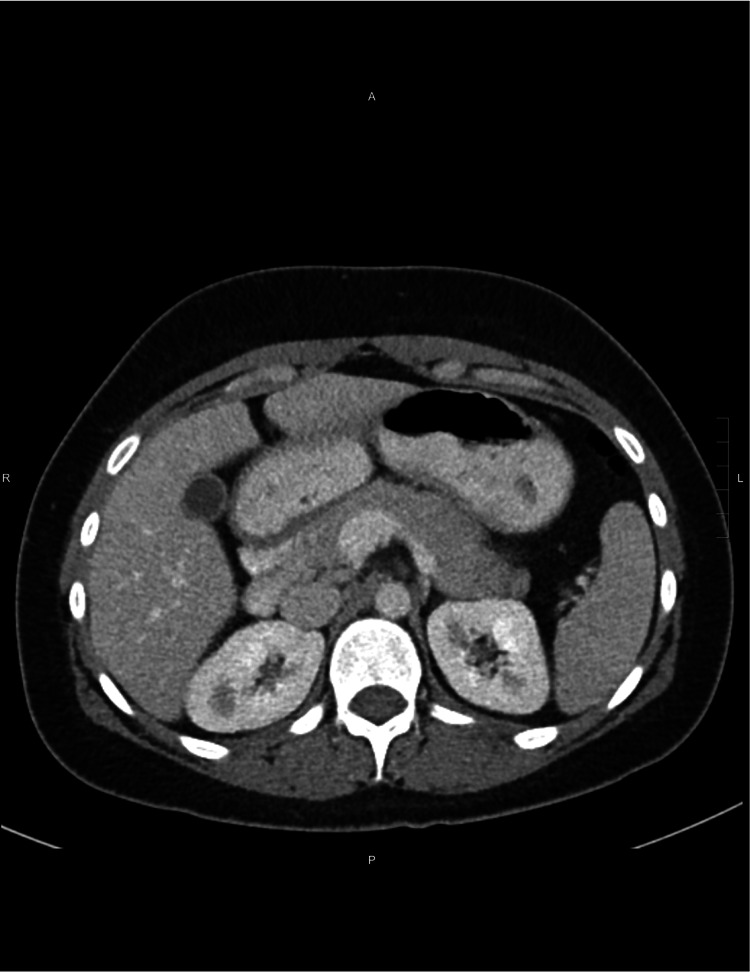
Repeat CT in two months shows resolution of previously identified small bowel wall thickening.
